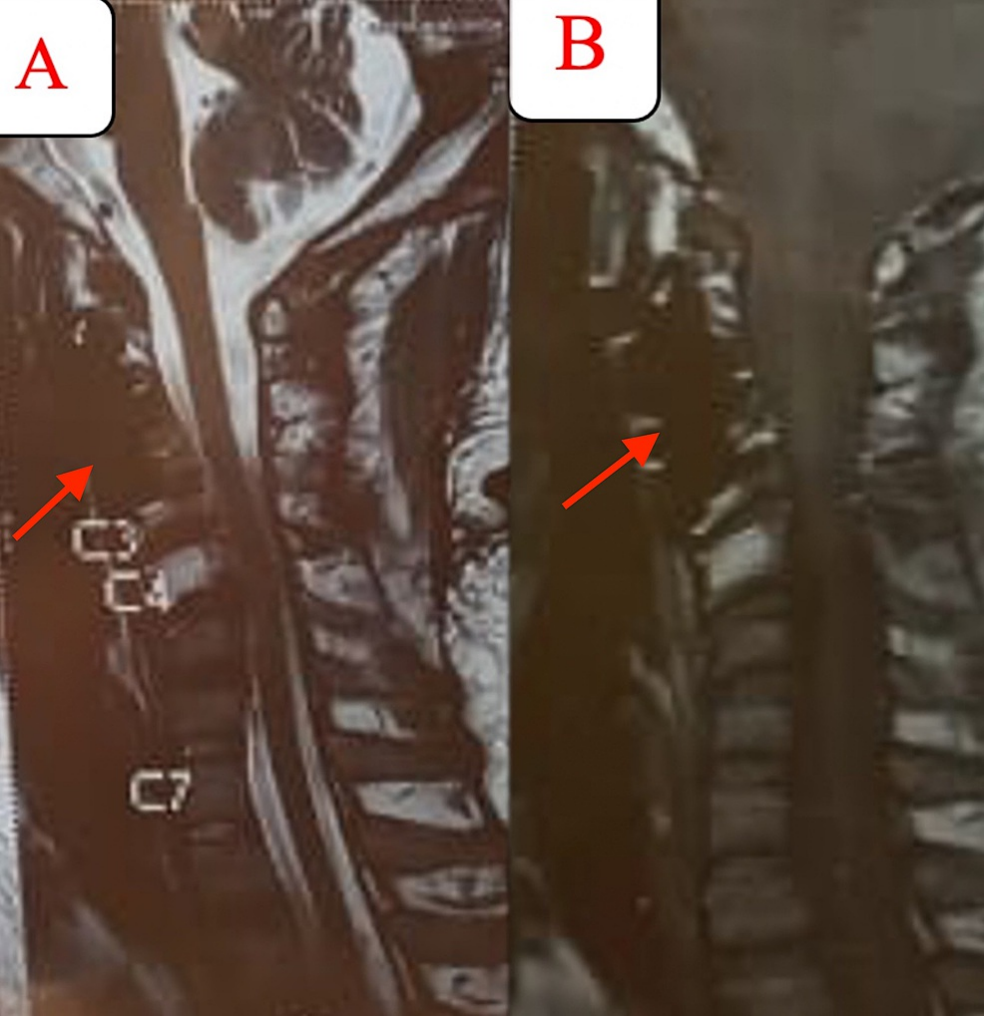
One year after the revision surgery, T2 (A) and T1 (B) sagittal MRI showed resolution of the tumor with spinal canal clearance. MRI: Magnetic Resonance Imaging
